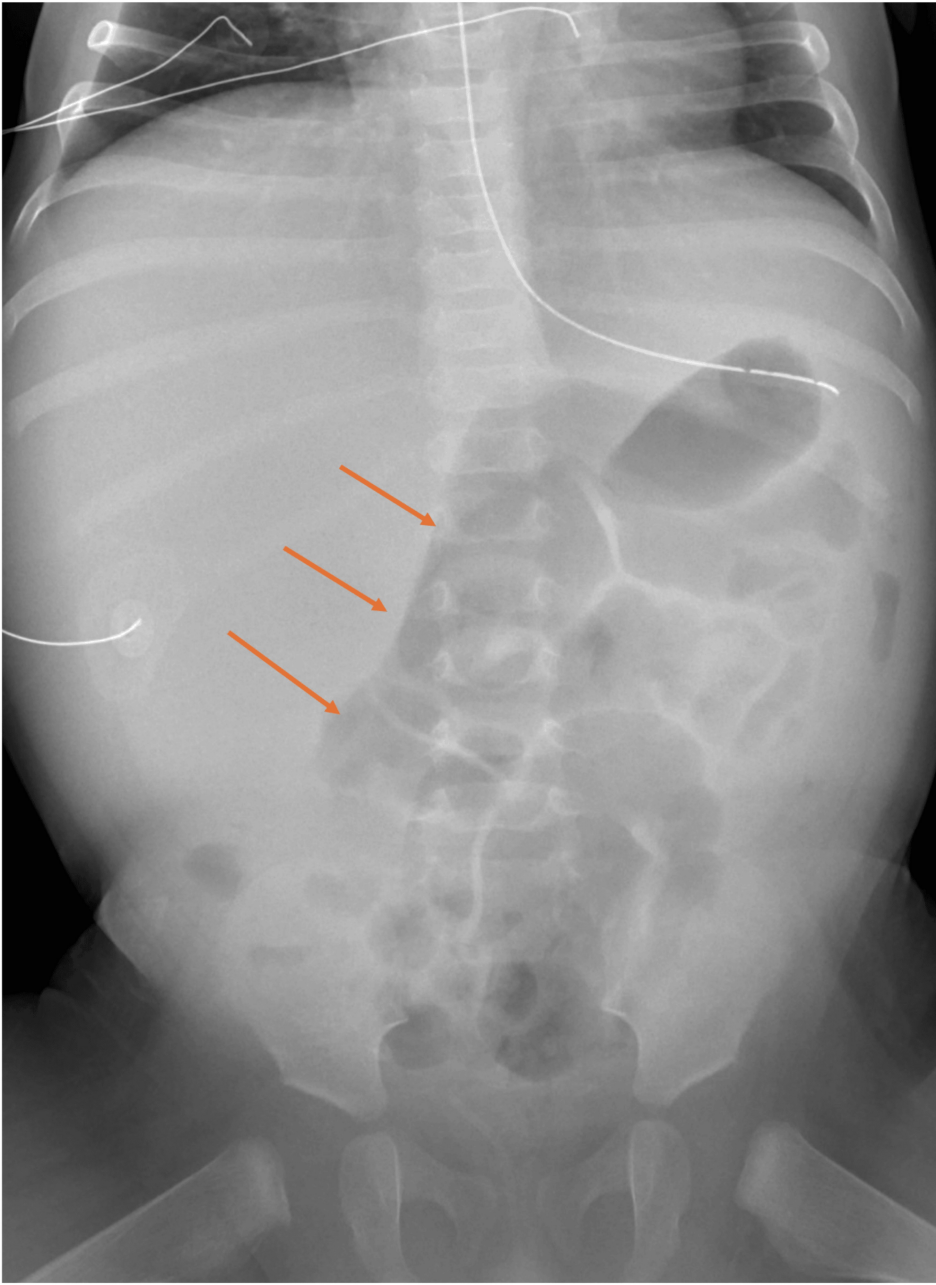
Supine Abdominal radiograph demonstrates displacement of the bowel loops to the center and left abdomen (orange arrows)
